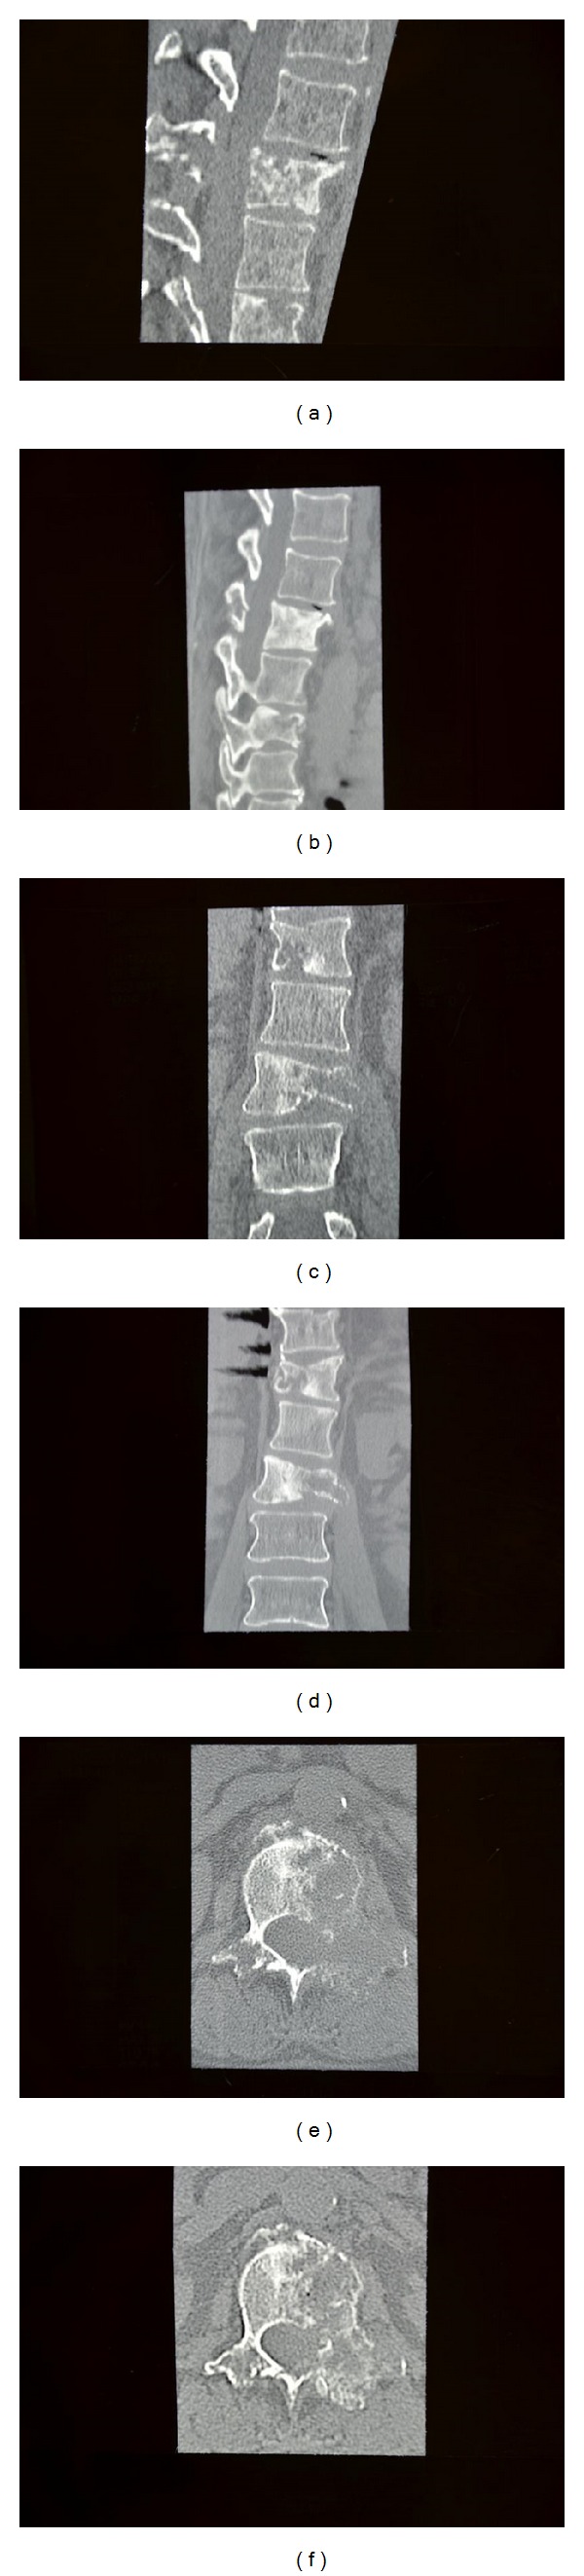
Case  1—computed tomography of thoracolumbar spine, noncontrast, demonstrating the development of bone remodeling and sclerosis 11 weeks after the initiation and 2 weeks after the completion of IAC (the vertebral body with maximal compression is L1). Sagittal before (a) and after (b), coronal before (c) and after (d), and axial L1 level before (e) and after (f) therapy.
